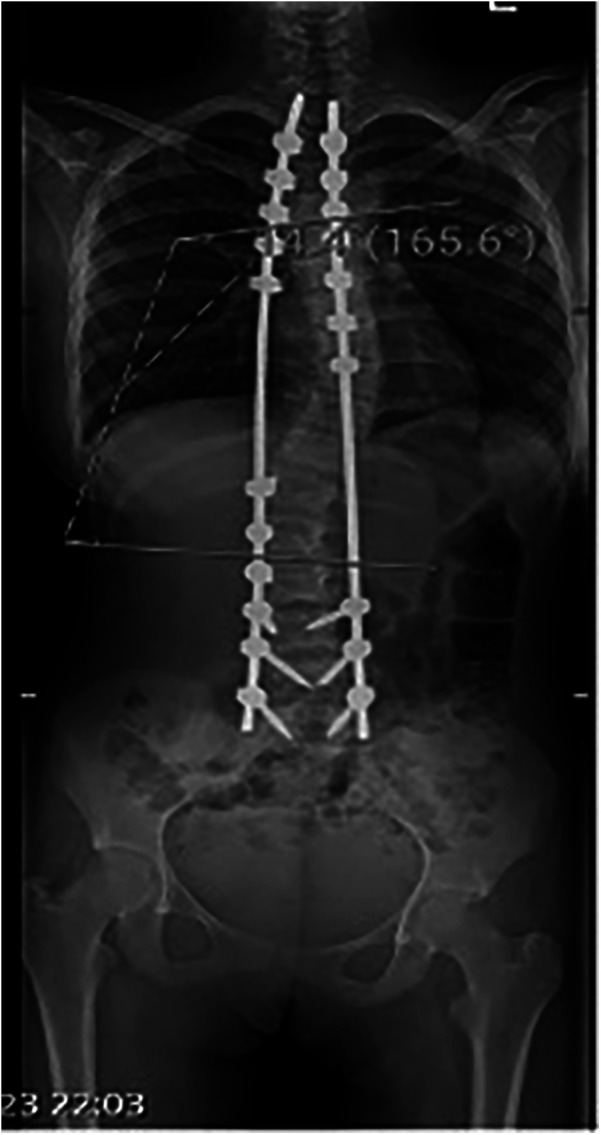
Postoperative anteroposterior radiograph demonstrating instrumented spinal fusion with satisfactory correction of coronal deformity.
